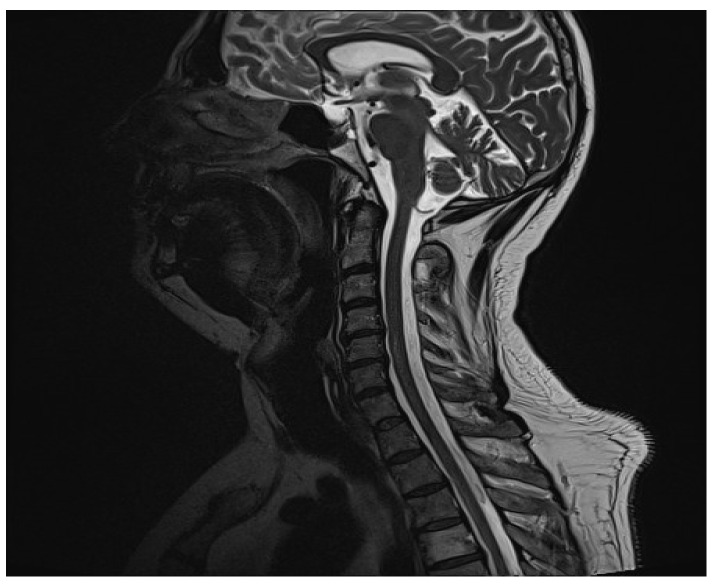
The MRI of the cervical spine on the T2-weighted short tau inversion recovery scan showed a hyperintense lesion on C5–C6.
